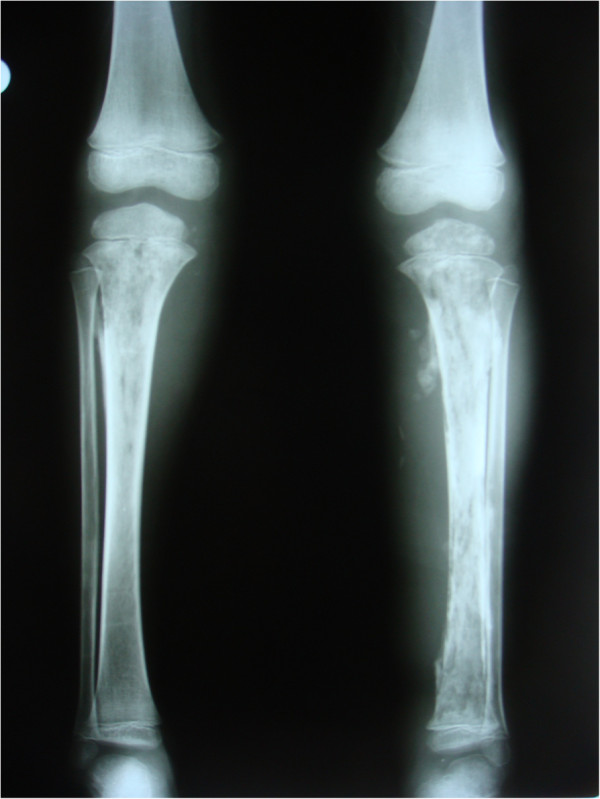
Anteroposterior radiograph of both tibia showing periosteal reactions and cortical destruction.
